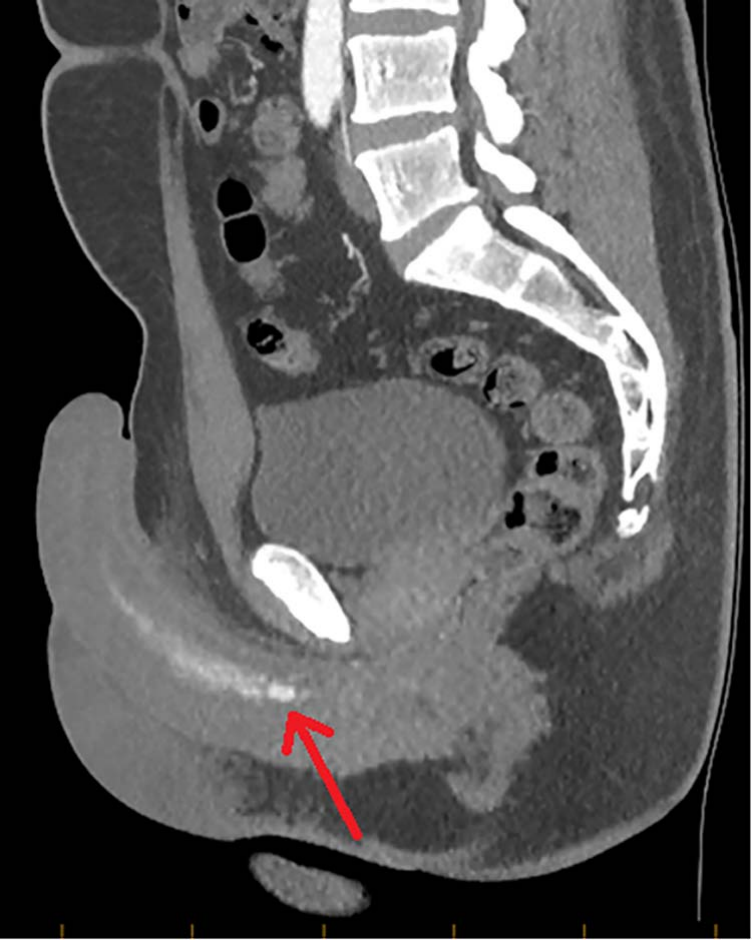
CTA demonstrating a sagittal view of contrast enhancement within the region of the left cavernosal artery and mid-to-distal corpus cavernosum (red arrow).
